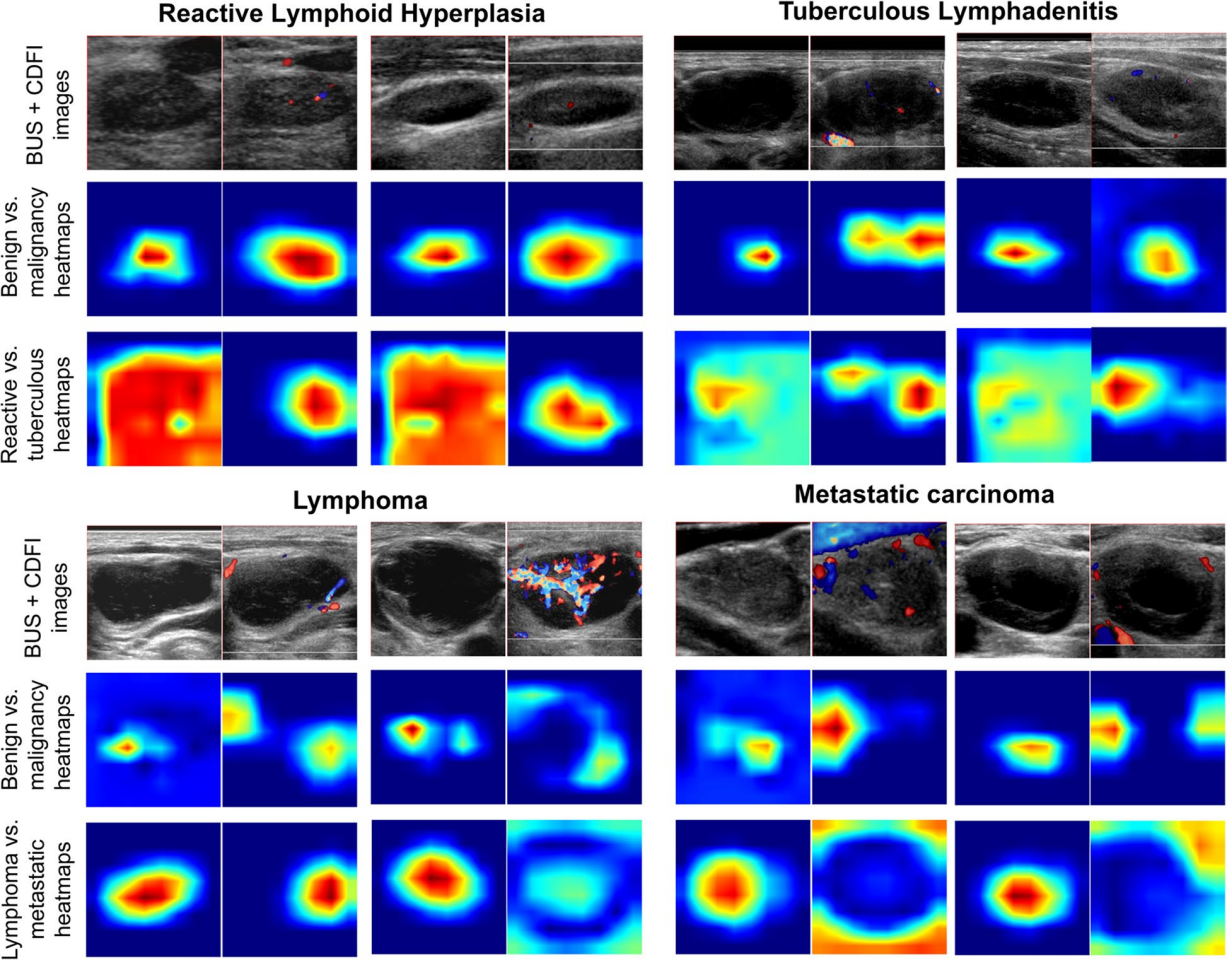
Examples of heatmaps generated by CLA-HDM for each etiology of unexplained CLA. When ultrasound BUS and CDFI images of a case (first row) are input into CLA-HDM, it will firstly give first-level diagnostic heatmaps to distinguish benign from malignant CLA (second row) and then second-level diagnostic heatmaps to identify the specific etiologies of benign or malignant CLA (third row). Generally, the heatmaps reveals a corresponding regularity for each pathology category. CLA, cervical lymphadenopathy; BUS, B-mode ultrasound; CDFI, color Doppler flow imaging
